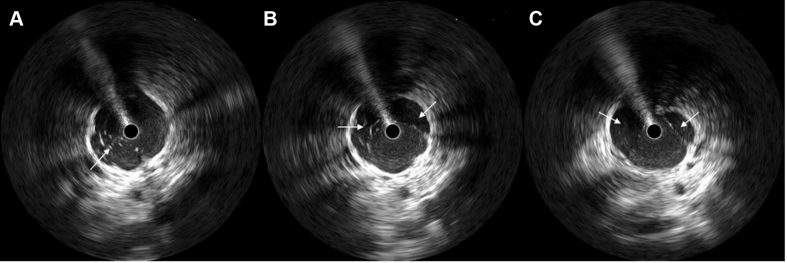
Intravascular Ultrasound of the Infrahepatic Inferior Vena Cava (A to C) Intravascular ultrasound (IVUS) imaging reveals multiple septate membranes (white arrows) within different segments of the infrahepatic inferior vena cava (the circle in the center of the vessel indicates the IVUS catheter).
